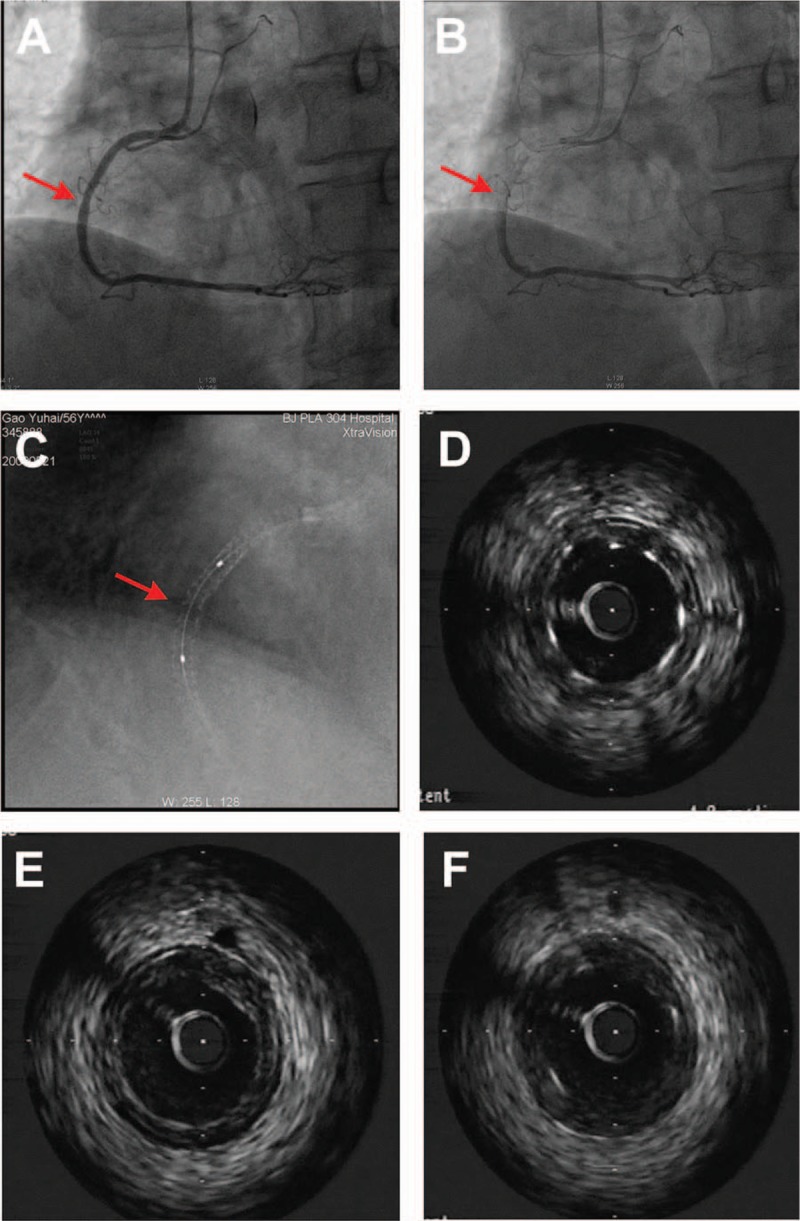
A, B, Coronary angiography showed 30% mild restenosis in the old stent of RCA (red arrow). C, StentBoost showed stent fracture in the place of mild restenosis in RCA (red arrow). D–F, IVUS images revealed mild neointimal hyperplasia in stents and the local absence of metallic stent struts (E). IVUS = intravascular ultrasound, RCA = right coronary artery.
